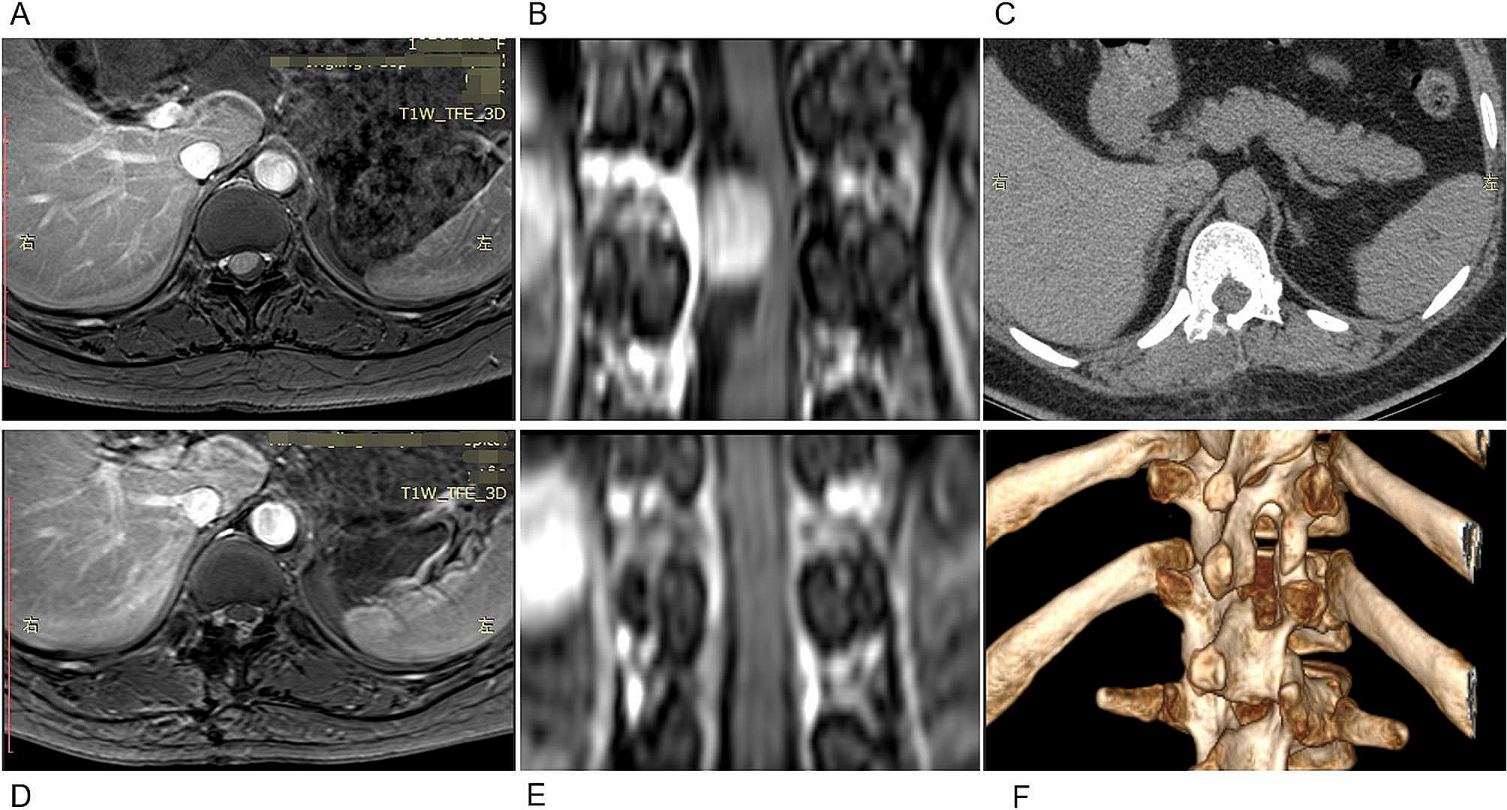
Endoscopic surgery through a tubular retractor for thoracic intradural schwannomas. A and B: Preoperative axial (A) and coronal (B) T1-weighted contrast-enhanced MR images suggest a T12 intradural-extramedullary schwannoma. C, D, E and F: Postoperative axial (D) and coronal (E) T1-weighted contrast-enhanced MR images demonstrating GTR with limited right hemilaminectomy, as shown on the postoperative axial CT scan (C) and 3D CT reconstruction (F)
